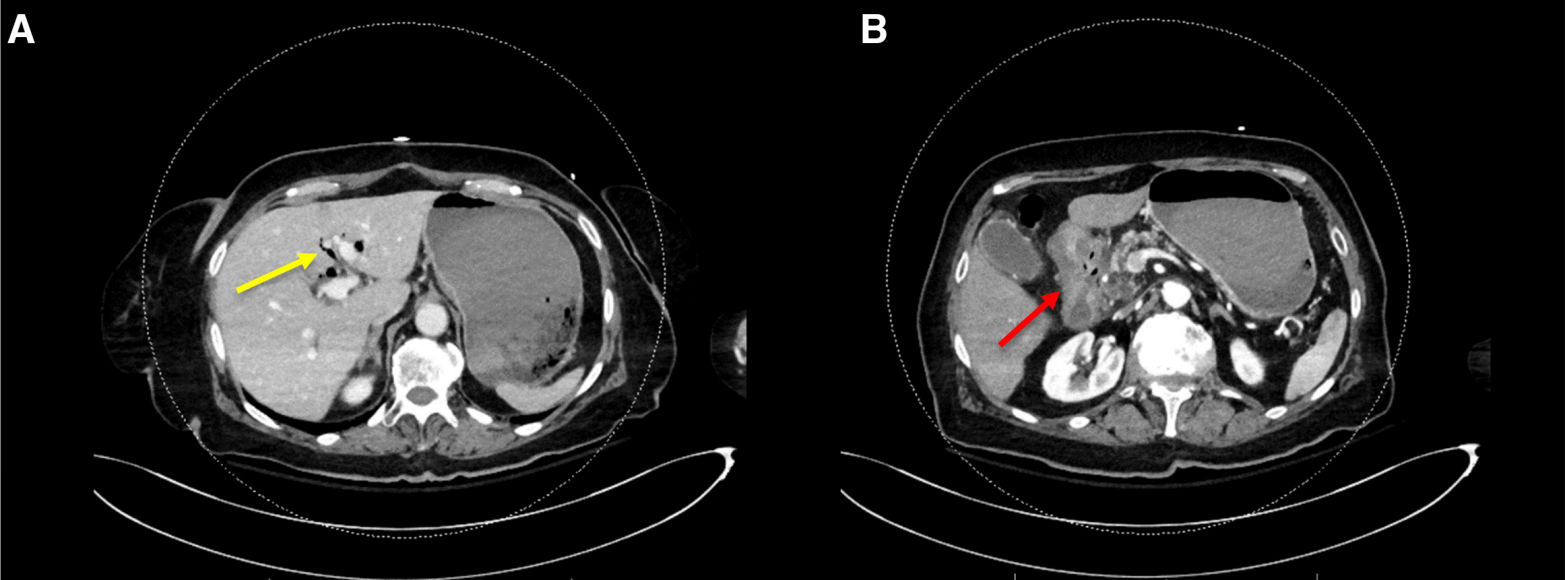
CT-scan of the abdomen showing (A) the presence of blood in the stomach, aerobilia (yellow arrow) and (B) inflammation of the first part of duodenum (red arrow).
